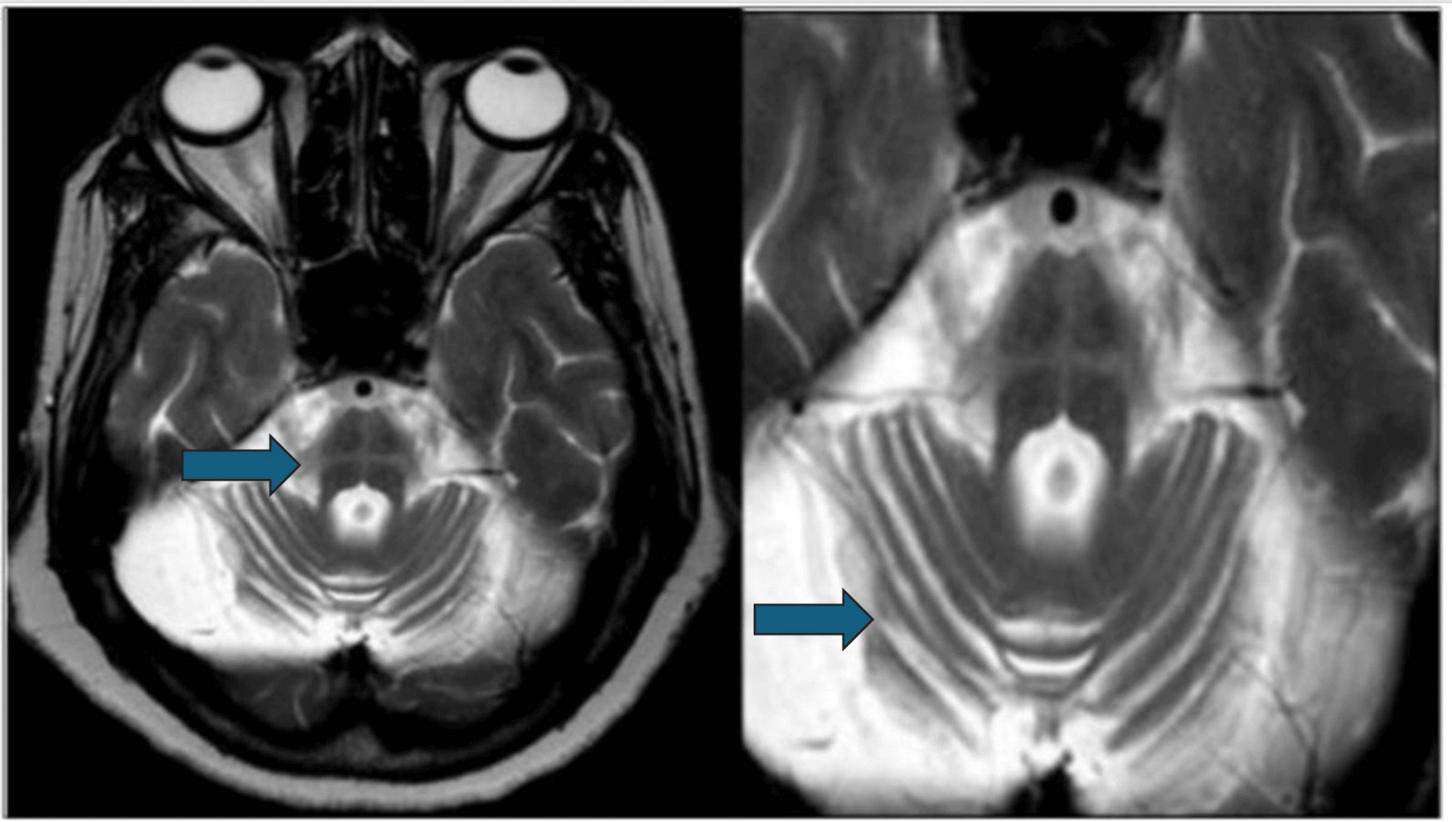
T2W axial images at the level of the superior cerebellar peduncle showing cruciate hyperintensity in the pons (hot cross bun sign) in MSA-C. The arrow in the first image is pointing toward cruciform hyperintensity in pons (hot cross bun sign). The arrow in the second image is pointing toward severe cerebellar atrophy in MSA-C. MSA-C = multiple system atrophy-cerebellar; T2W = T2-weighted
